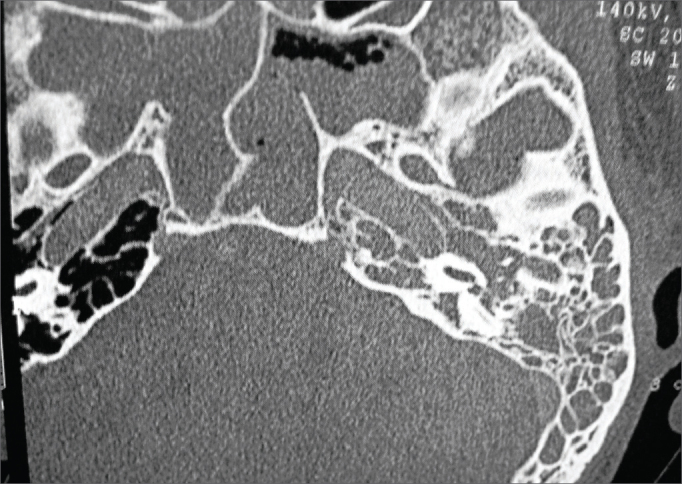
Temporal bone CT scan, axial view showing matter with soft tissue density on the mastoid and in the left middle ear and sphenoidal sinus.
